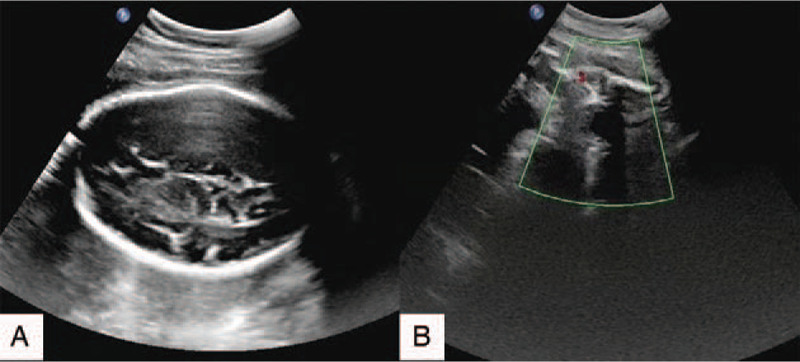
Ultrasound showed a hypoechoic mass behind the uterus, about 23.0 × 12.5 cm in size.
